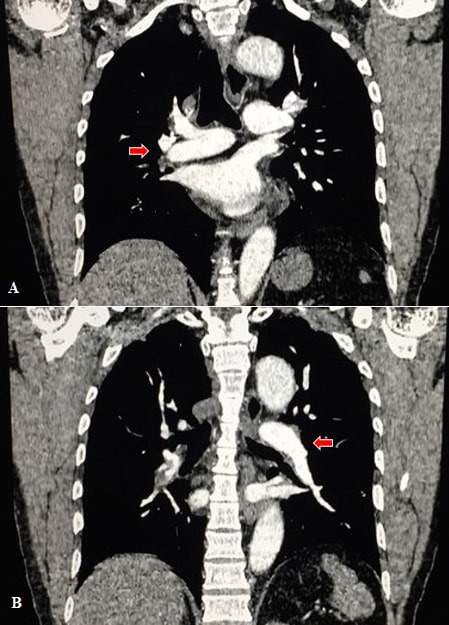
Computed tomography angiography of the chest, coronal plane, showing restored blood flow to right (A) and left (B) pulmonary arteries and removal of a large proportion of the thrombus.
